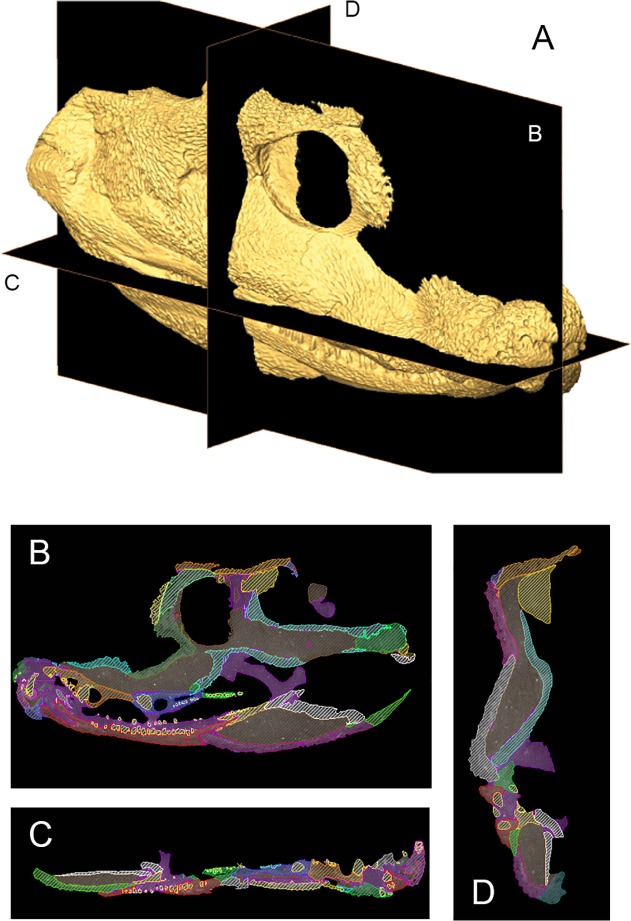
Segmentation of CT data of *Acanthostega gunnari*. Isosurface (A) of (MGUH-VP-8160) with labeled sagittal (A), frontal (B) and transverse (C) planes. These slices are shown in insets, with shaded areas representing individual bones segmented from each other and the surrounding matrix.
